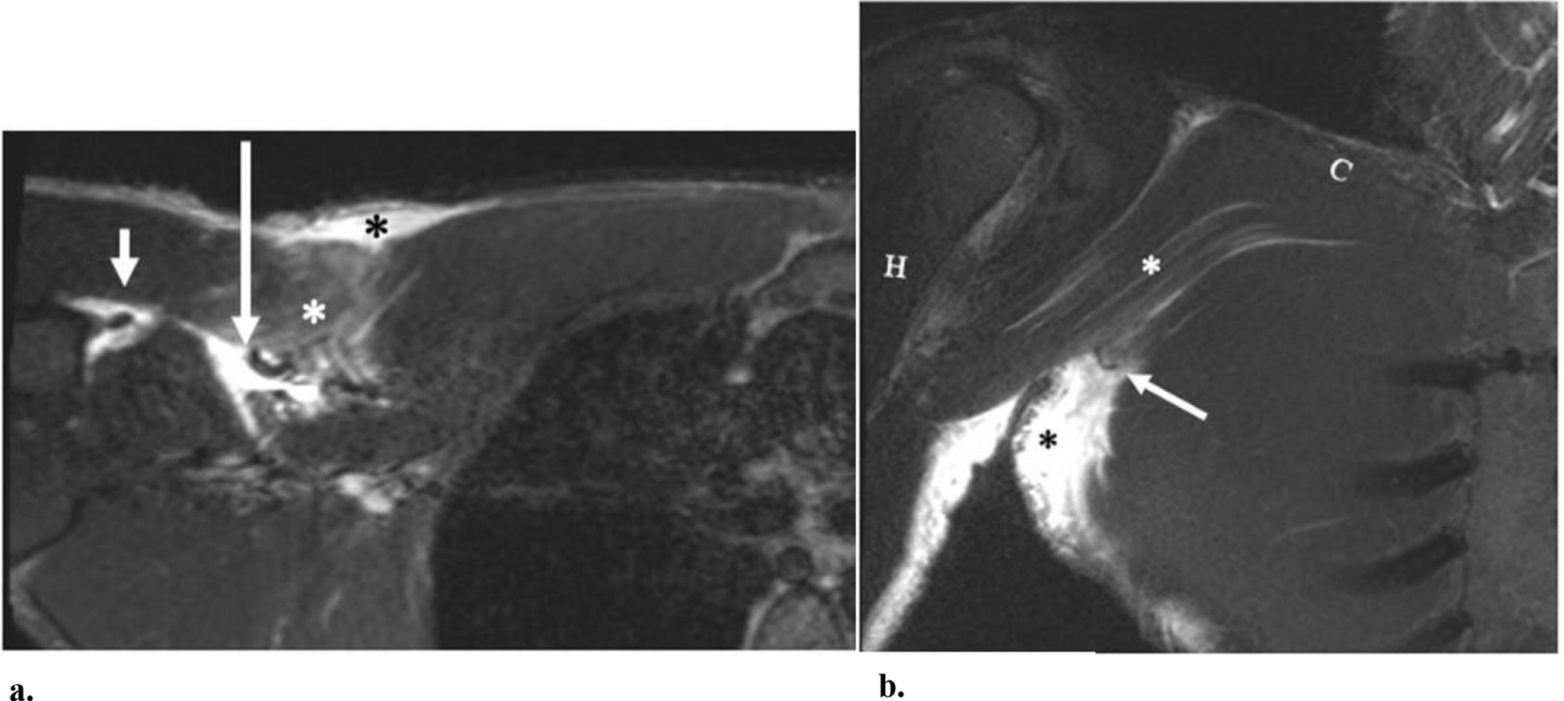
42-year-old-male that presented after feeling a pop in the anterior chest when bench-pressing. (a) Axial STIR MR image shows a full-thickness, partial-width tear with a medially retracted tendon stump (long arrow) originating from the sternal head of the pectoralis major with areas of intramuscular edema (white asterisk) related to sites of interstitial tears. A triangular peri-bicipital hematoma (short arrow) is shown lateral to the retracted tendon stump. Anterior subcutaneous edema is also present (black asterisk). (b) Coronal STIR MR image also demonstrates the retracted tendon stump (arrow) with regional edema (black asterisk). Intramuscular edema is present along the intact clavicular fibers of the pectoralis major (white asterisk). The shafts of the clavicle (C) and humerus (H) are also denoted. At surgery, there was a complete tear of the sternal head from its humeral attachment with medial retraction and extension of the tendon rupture into the myotendinous junction and muscle belly
